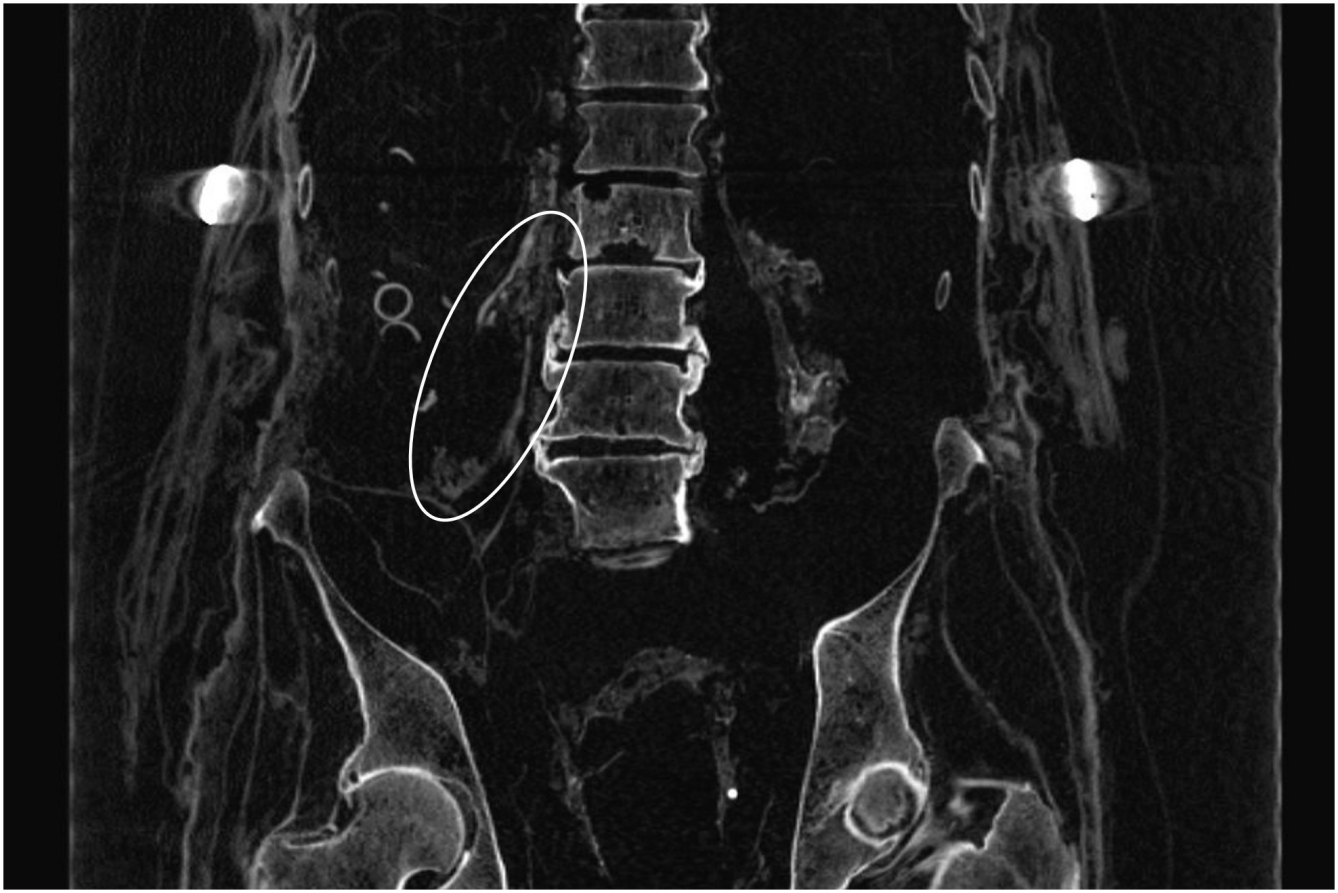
Coronary plain section of the CT scans with a further aspect of the cystic lesion at the retroperitoneal space (circle).
